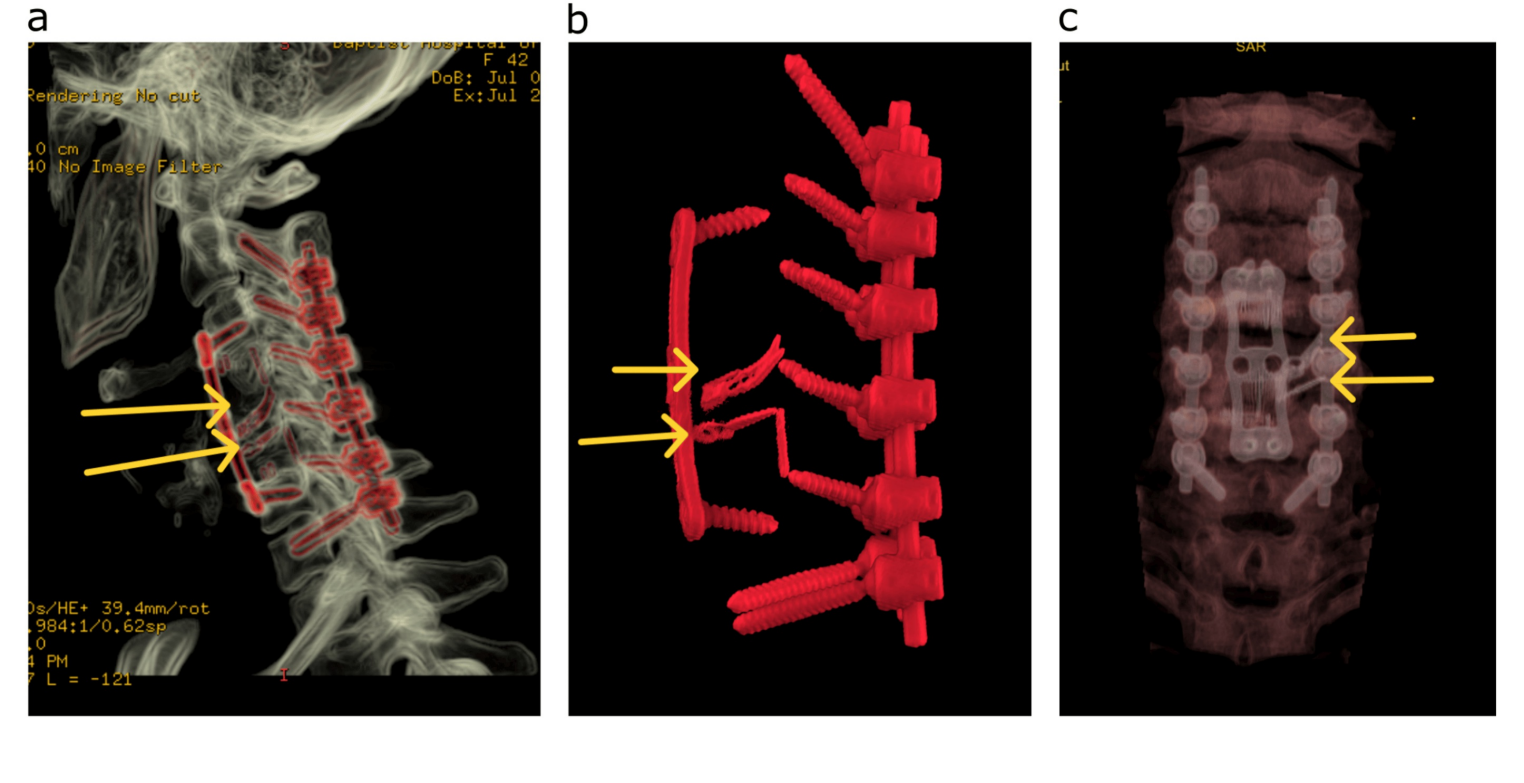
Post-operative CT reconstructions (a) Post-operative CT scan reconstruction (yellow arrows with aneurysm clips). (b) Subtracted reconstruction demonstrating the intra-operative modification of the PEEK interbody cage and accommodating the aneurysm clips (yellow arrows) on the sacrificed left vertebral artery. (c) Coronal reconstruction showing the construct (yellow arrows to aneurysm clips). CT: computed tomography; PEEK: polyetheretherketone
